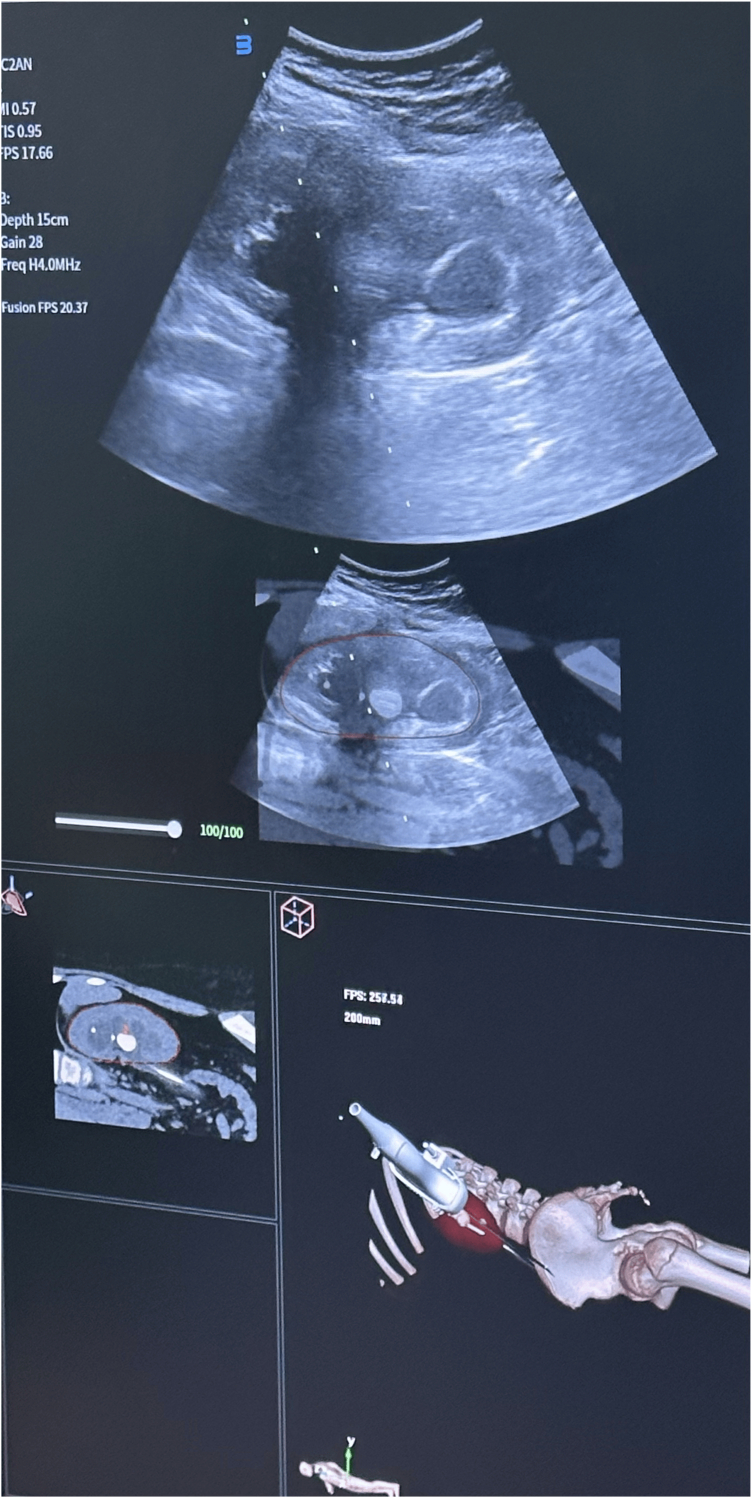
Intraoperative view of the computed tomography–ultrasound fusion technique.
